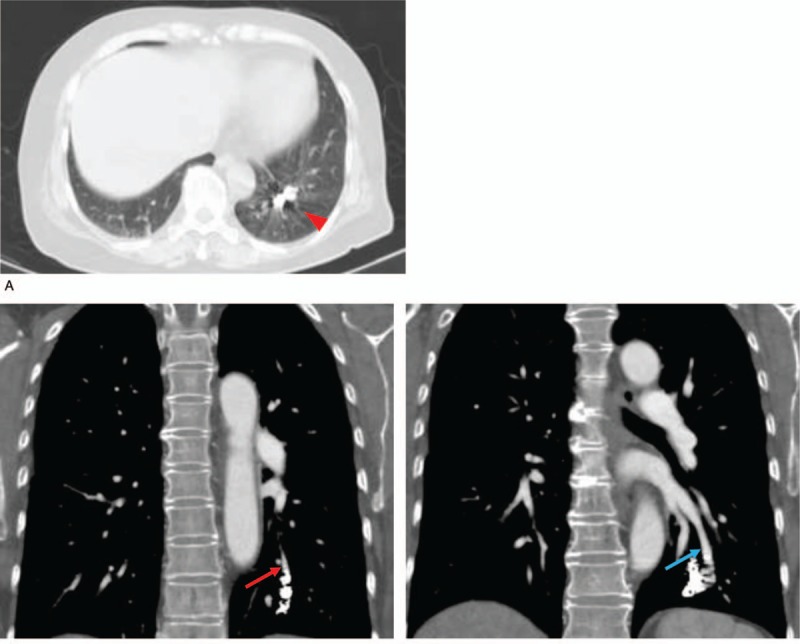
(A) Computed tomography (CT) revealed that the coil had been placed in the lower lobe S10 of the left lung in 2004 (arrow head). (B) Contrast CT revealed a contrast effect in the inflow (red arrow) and outflow (blue arrow) blood vessels of the pulmonary arteriovenous malformation (PAVM) in the lower lobe S10 of the left lung.
